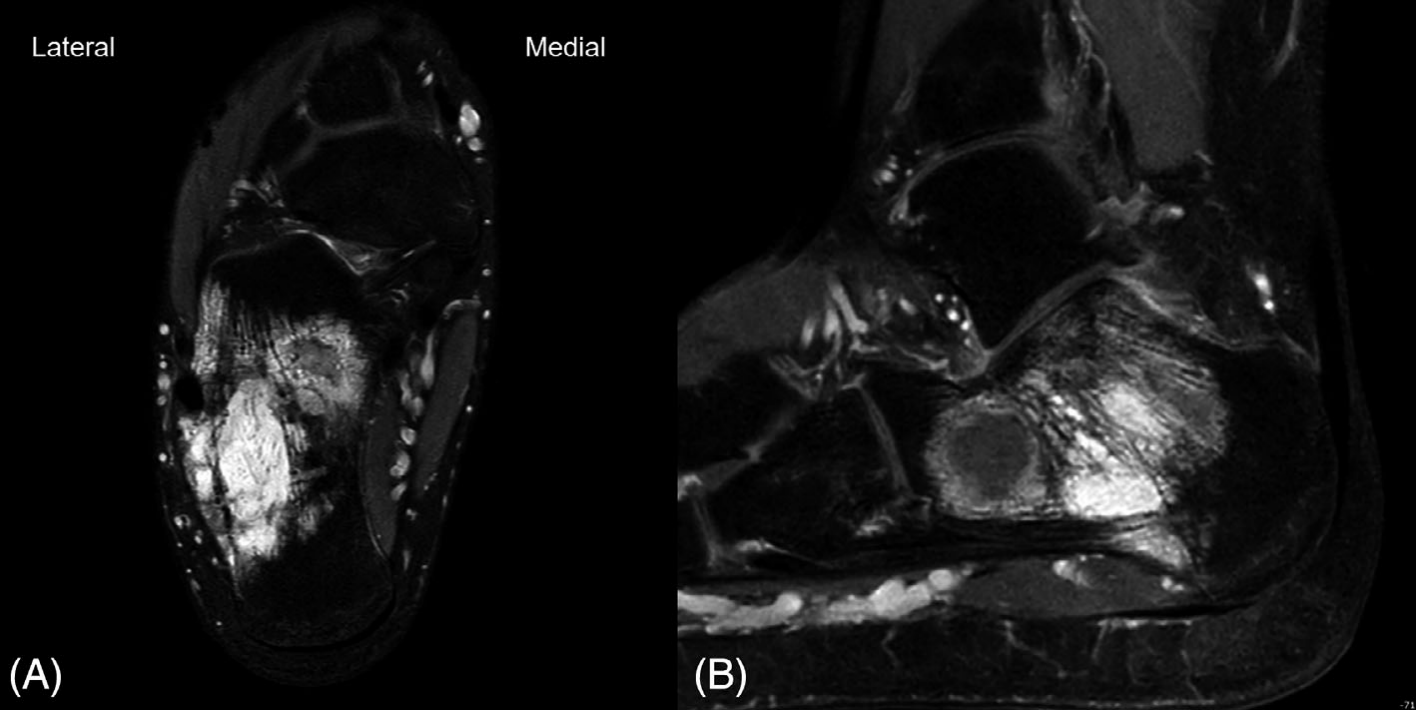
Magnetic resonance imaging with a calcaneus bone lesion with soft tissue component, very vascular, that breaks the bone cortex, with necrosis focus inside. (A) Coronal view. (B) Sagittal view
